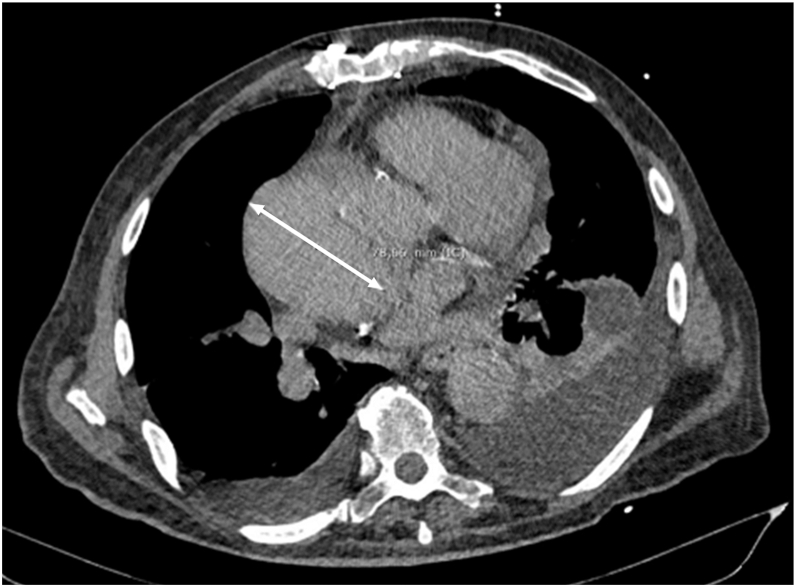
Transaxial computed tomography scan image showing an ascending aortic aneurysm of 7.8 cm.
